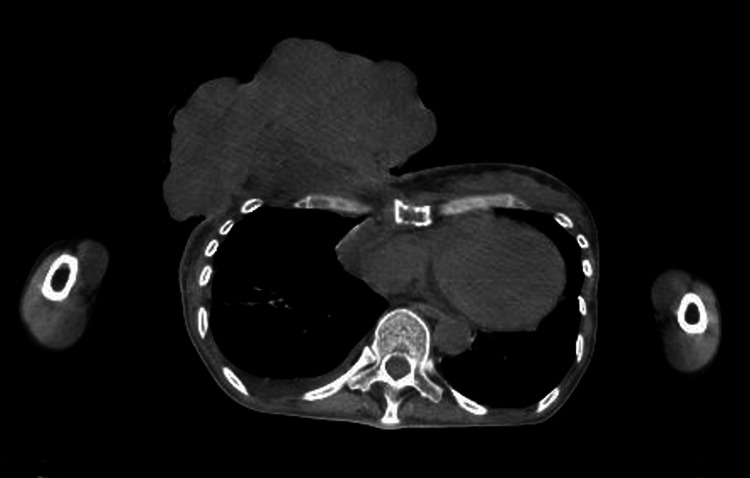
Axial enhanced computed tomography images. Images of the thoracic region show a tumor that had spread from the right chest wall to the outside of the skin. The lesion had not invaded the rib bone. Slight pleural effusion was observed in the right lung.
